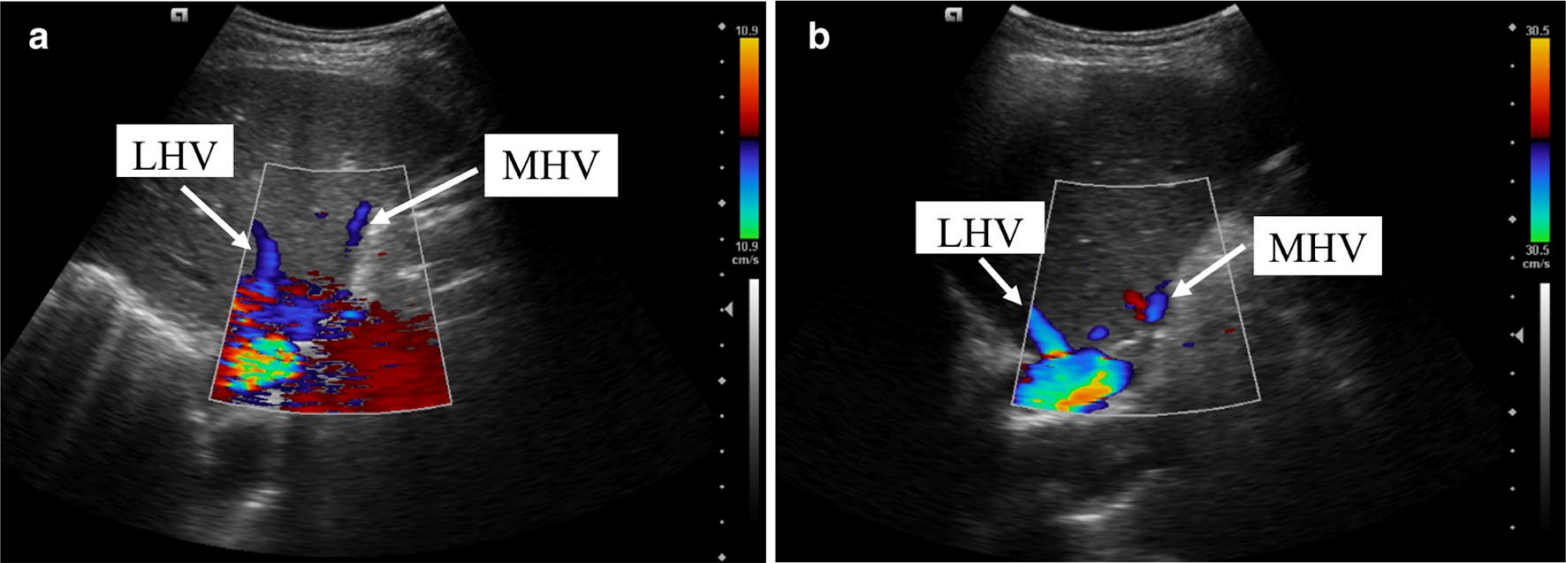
Ultrasound on postoperative day 4 (a) and postoperative day 60 (b). *MHV* middle hepatic vein, *LHV* left hepatic vein
